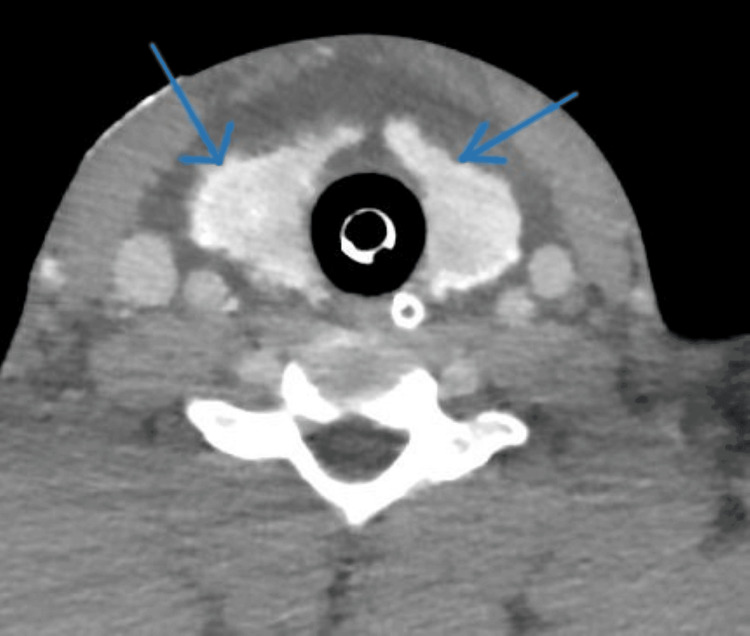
Axial contrast-enhanced CT of the neck demonstrating shock-related thyroid change. Contrast-enhanced CT scan of the neck in axial view (venous phase; slice thickness 5 mm; window/level 350/50 HU) demonstrating bilateral perithyroidal edema and simple fluid (blue arrows) surrounding a normally enhancing thyroid gland, characteristic of shock-related thyroid change. The trachea (Tr) and strap muscles (SM) are visualized for orientation. HU: Hounsfield unit
